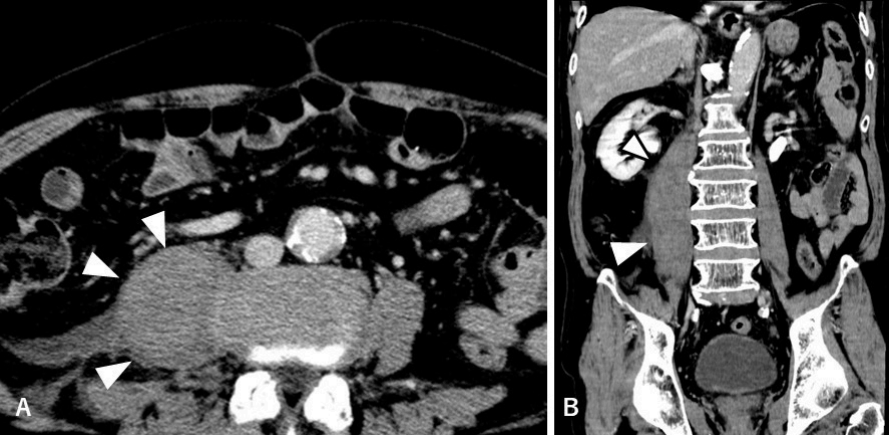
Swollen right iliopsoas muscle (white arrowheads) on contrast CT scan. (A) Horizontal view. (B) Coronal view.
